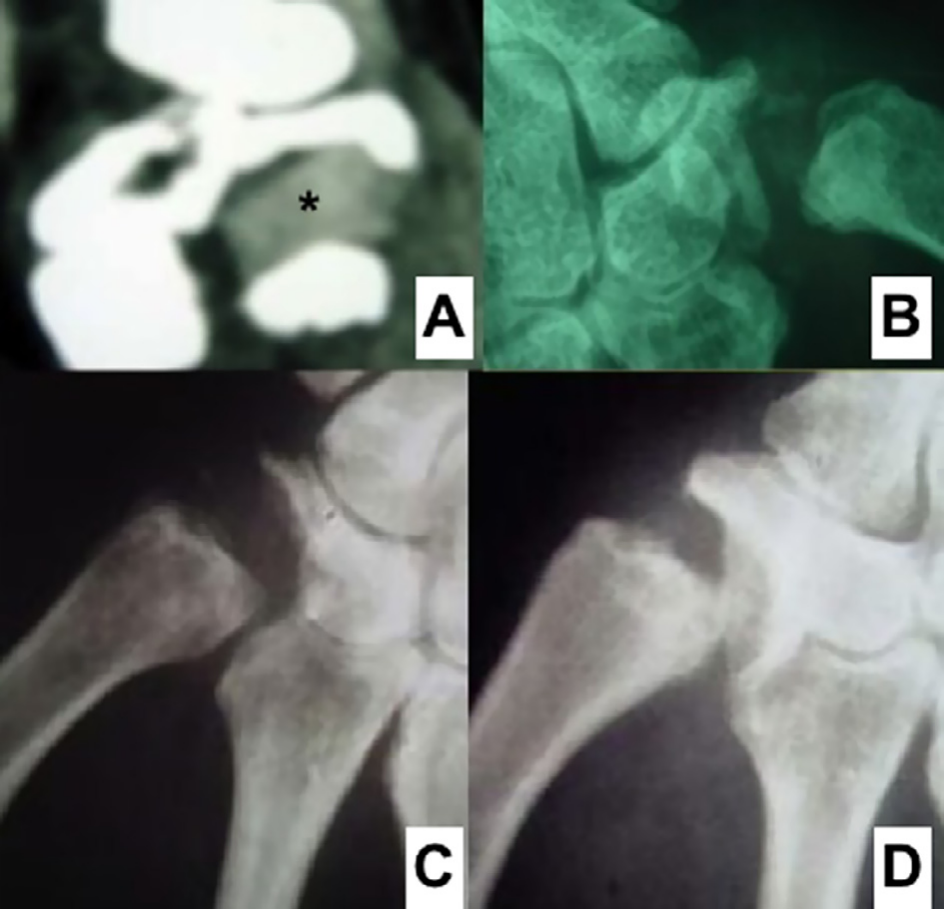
CT scan aspect of a graft (*) in the space created by trapeziectomy (A), slight metaplasia appeared sometimes with time without any clinical consequences (B), Narrowing of the space appeared with high follow-up but less than trapeziectomy alone (C & D).
